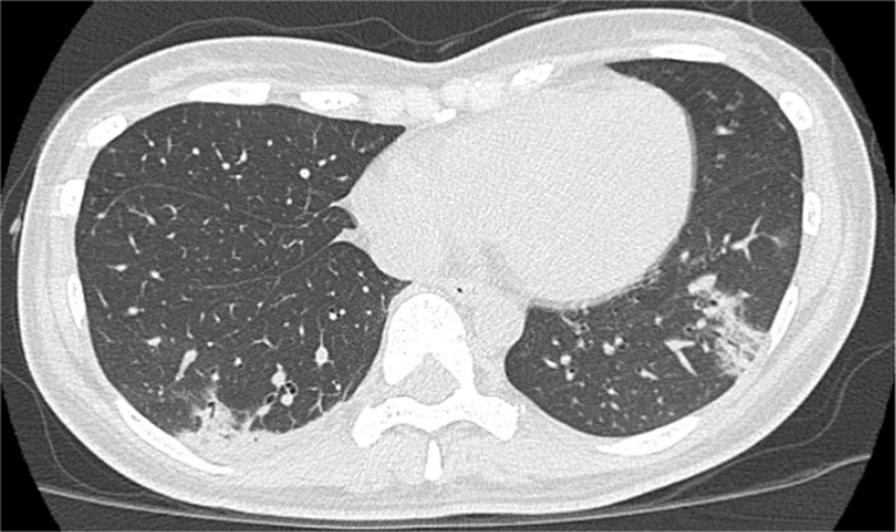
Chest CT findings. Chest CT findings at the diagnosis of COVID-19 showing ground-glass opacity in the peripheral lesion of both lungs
